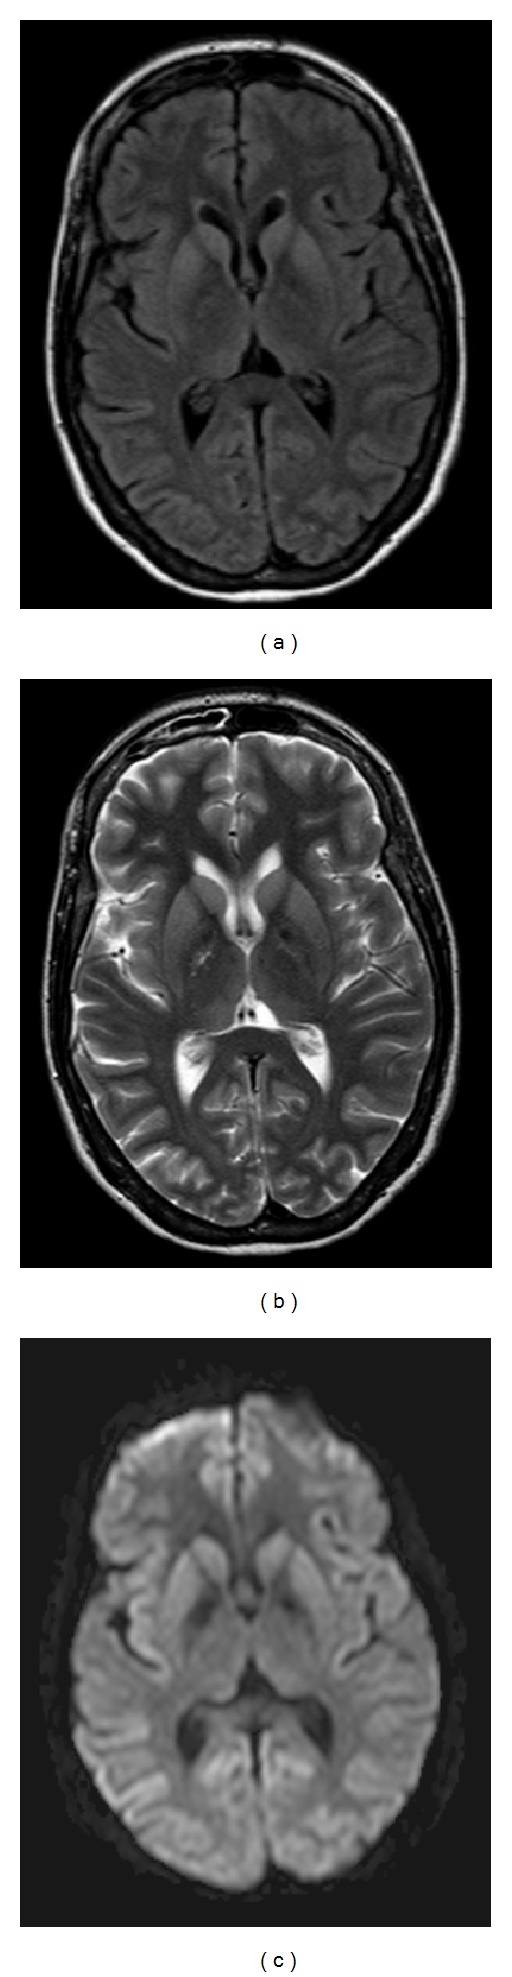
MRI findings (axial FLAIR, T2, and DWI). Subtle symmetrical hyperintensities in the caudate nucleus and putamen and less evident in the pulvinar nuclei and cerebral cortex are noticeable.The figure is constructed by J. Dorgelo.
